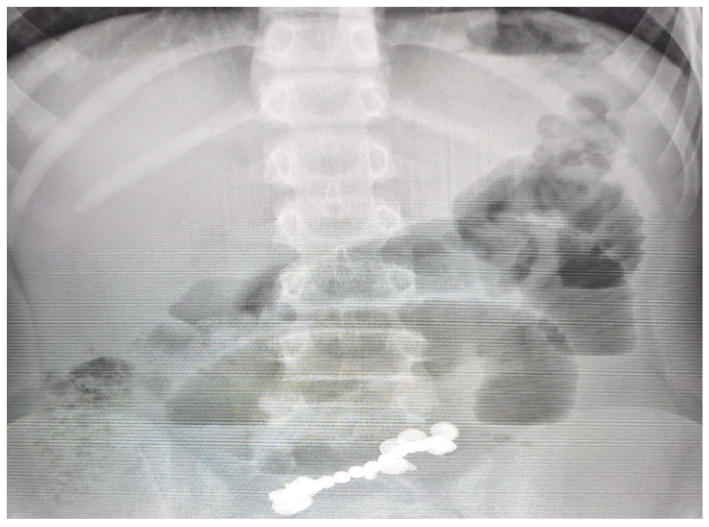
Plain abdominal radiograph demonstrating multiple radiopaque spherical foreign bodies clustered in the distal ileal region, consistent with ingested high-powered magnets. Associated bowel gas pattern suggests small bowel obstruction.
